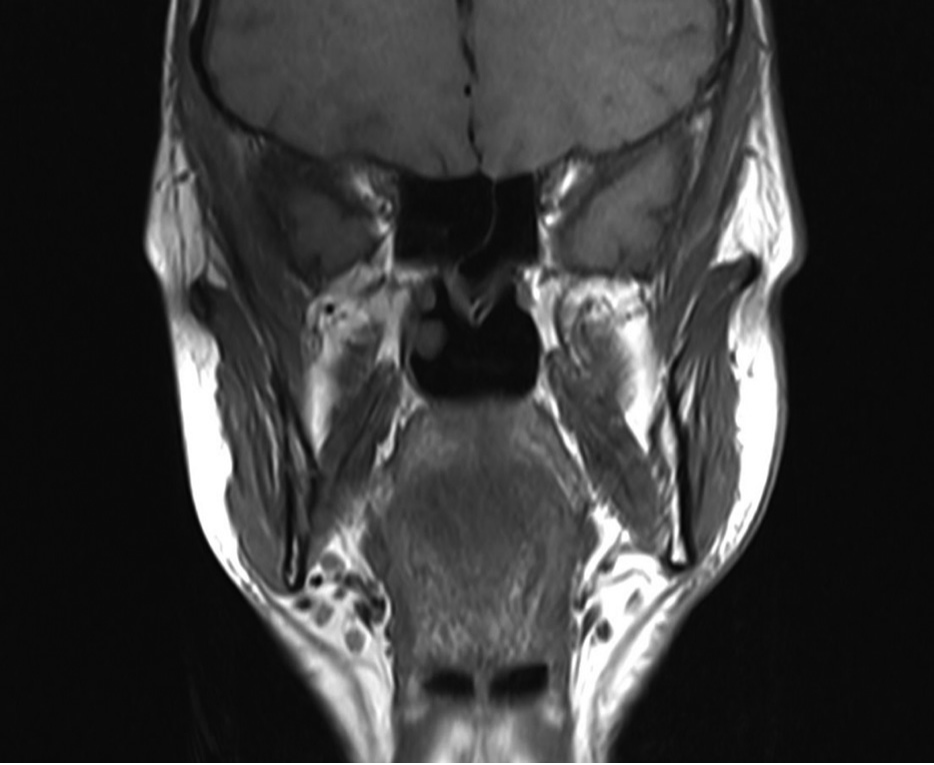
MRI revealing agenesis of all major salivary glands.
